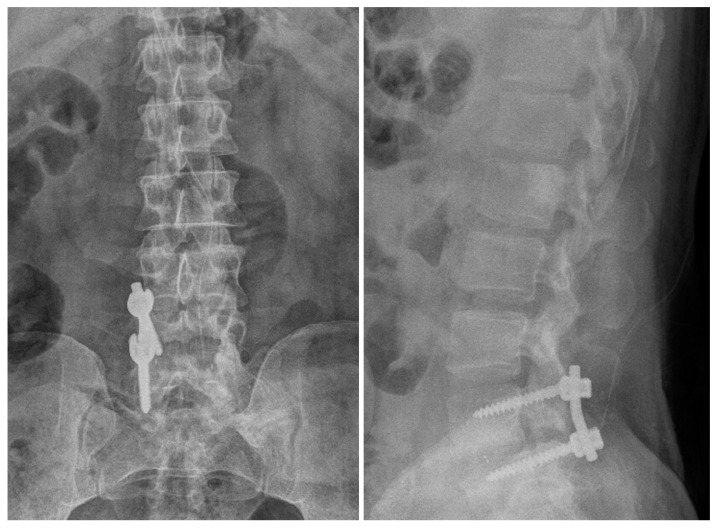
Radiographicimage after the aggressive decompression and fusion surgery at L4/L5.
